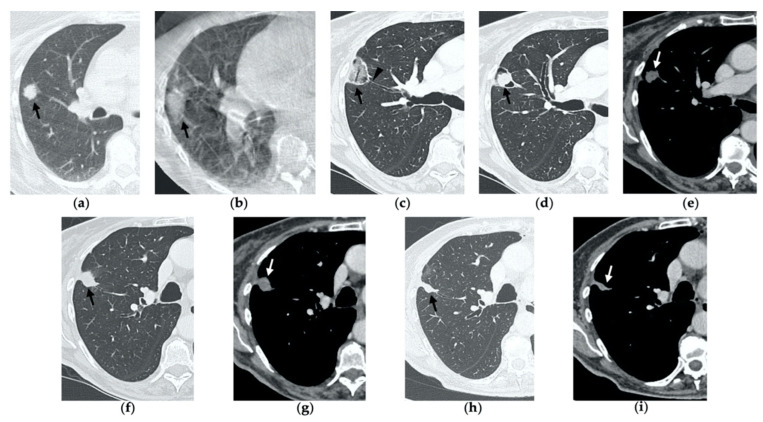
69-year-old woman with a biopsy-proved NSCLC in the right upper lobe. (a) Pre-treatment axial CT. (b) Cone-beam CT image post-MWA shows GGO (black arrow) among the ablation site. (c–e) Axial 1-month follow-up CT shows (c) a large consolidation with well-defined margins, inner hypoattenuating bubbles and a cavitation (black arrow) in communication with a peripheral bronchus (arrowhead); (d) on a different level, central necrotic material is seen within the cavitation (black arrow) (e) with no contrast enhancement (white arrow). (f,g) Axial 6-month follow-up CT images show resolution of the cavitation and decrease in size of the consolidation (black arrow) with no contrast material uptake (white arrow). (h,i) Axial 9-month follow-up CT image shows a linear fibrotic band with no contrast enhancement.
